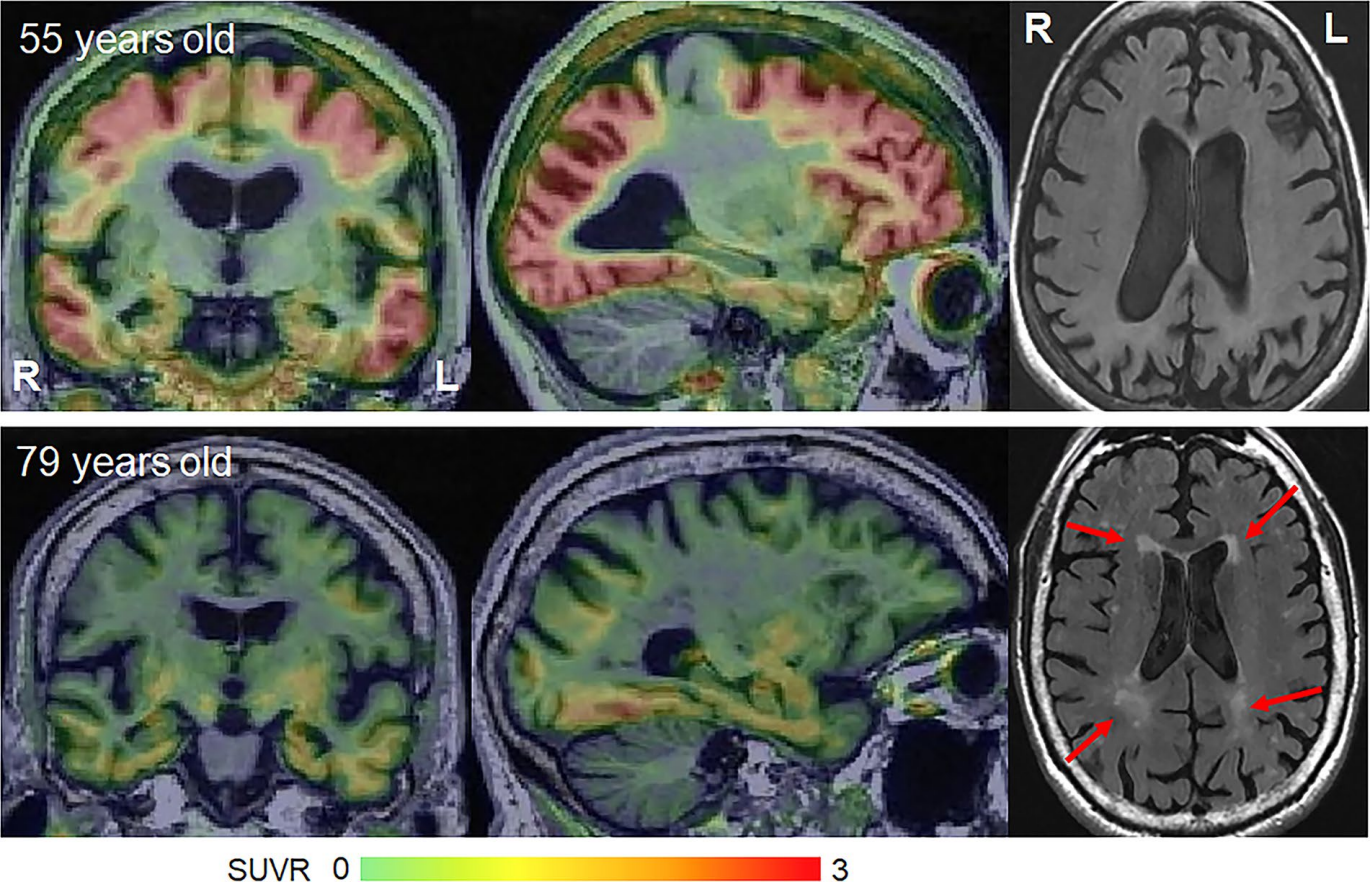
Flortaucipir PET uptake and white matter hyperintensities on FLAIR in young-onset and late-onset amnestic AD patients. The flortaucipir PET images are shown as standardized uptake value ratio (SUVR) images referenced to the cerebellar crus grey matter. The young-onset patient shows widespread flortaucipir uptake with no WMHs, while the late-onset patient shows milder flortaucipir uptake and moderate WMHs.
